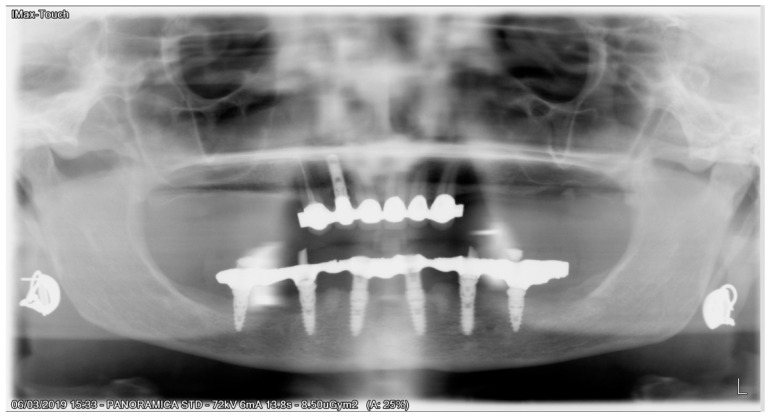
2 years postoperative panoramic X-ray.
